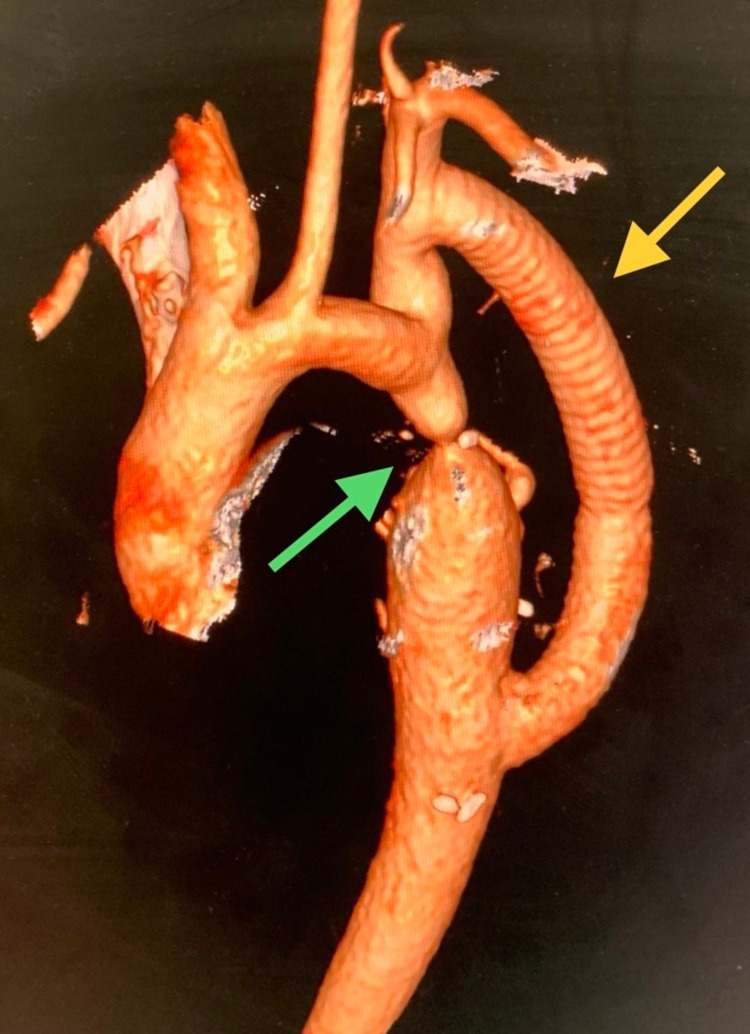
CT angiogram of the chest region, volume rendered image, showing left subclavian to descending thoracic aortic bypass (yellow arrow).The coarctation can also be seen (green arrow).
